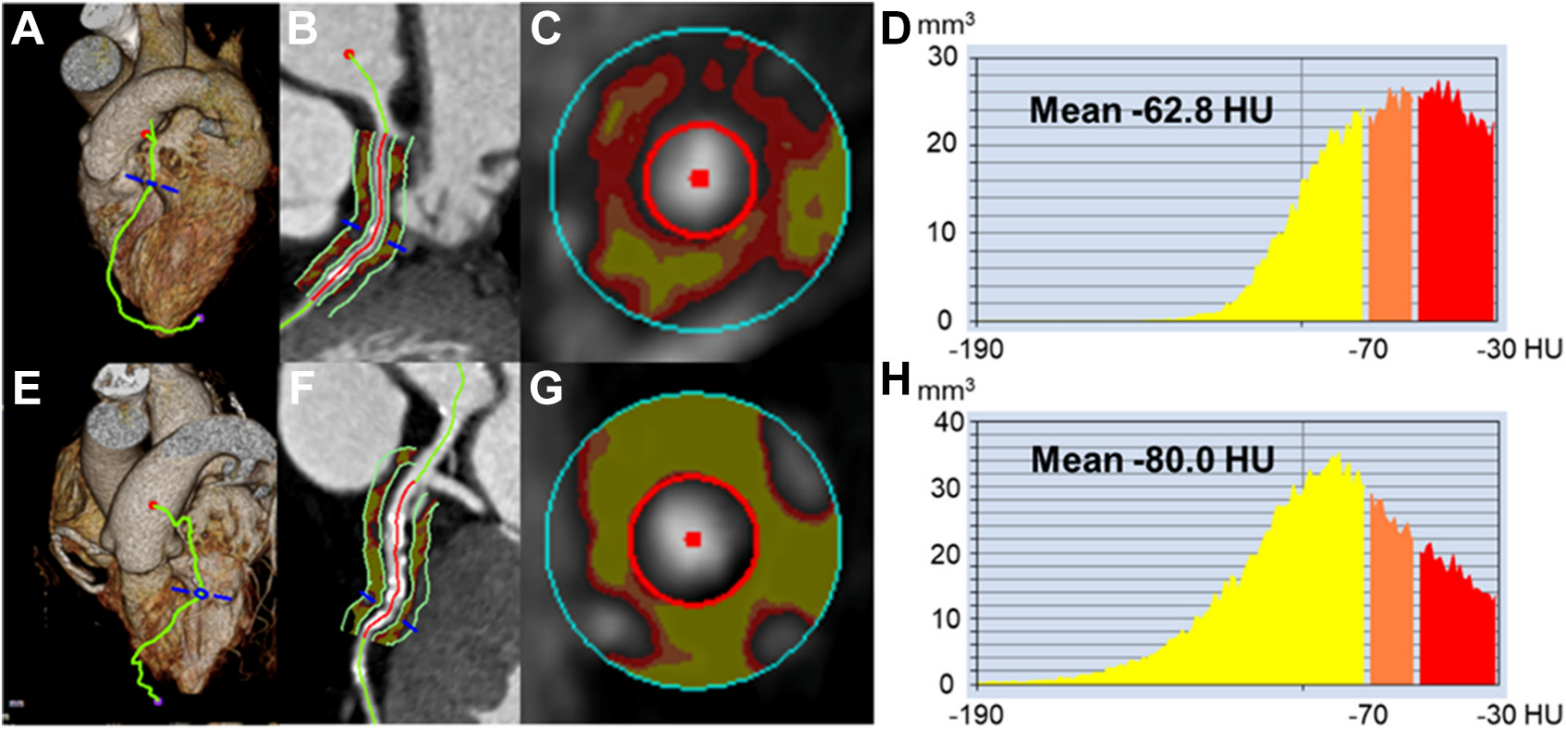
Representative Case Showing Fat Attenuation Index by Coronary Computed Tomography Angiography Three-dimensional reconstruction of the heart (A) and (E); pericoronary adipose tissue attenuation between −190 HU and −30 HU in the longitudinal view (B) and (F) and cross-sectional view (C) and (G); around the proximal 40 mm of the left anterior descending artery; histogram of CT attenuation within the traced area (D) and (H). A to D show pericoronary fat attenuation index in a case with the incidence of heart failure during follow-up. E to H show pericoronary fat attenuation index in a case without incidence of heart failure during follow-up. CT = computed tomography.
